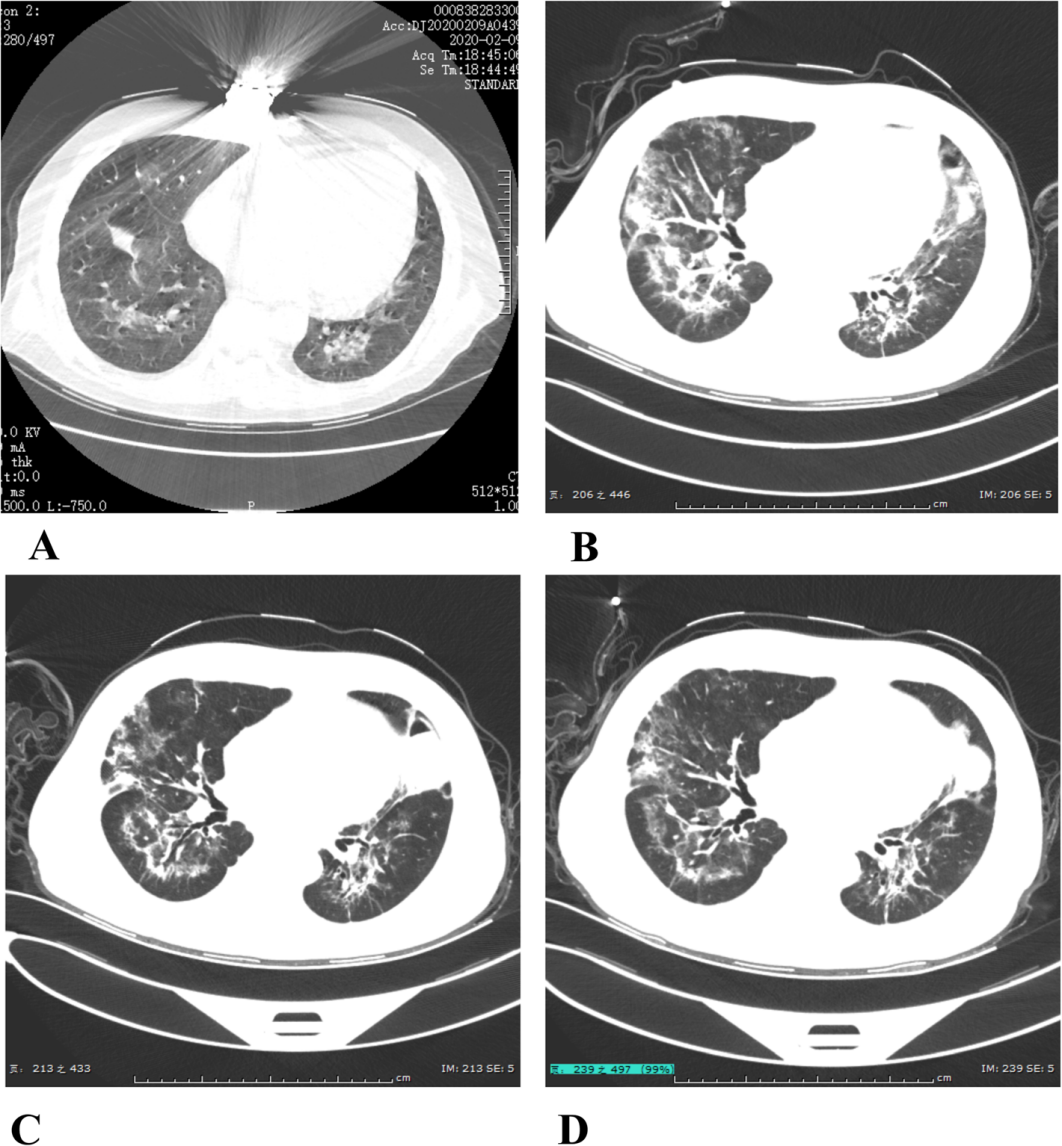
The chest computed tomography (CT) scan showed bilateral diffuse ground-glass changes and interstitial thickening on admission (a), aggravation (with large patchy opacities, local consolidation and pleural incrassation or conglutination) on day 10 (b), partial decrease of multiple patchy opacities on day 18 (c), and decrease of local consolidation on day 25 (d)
